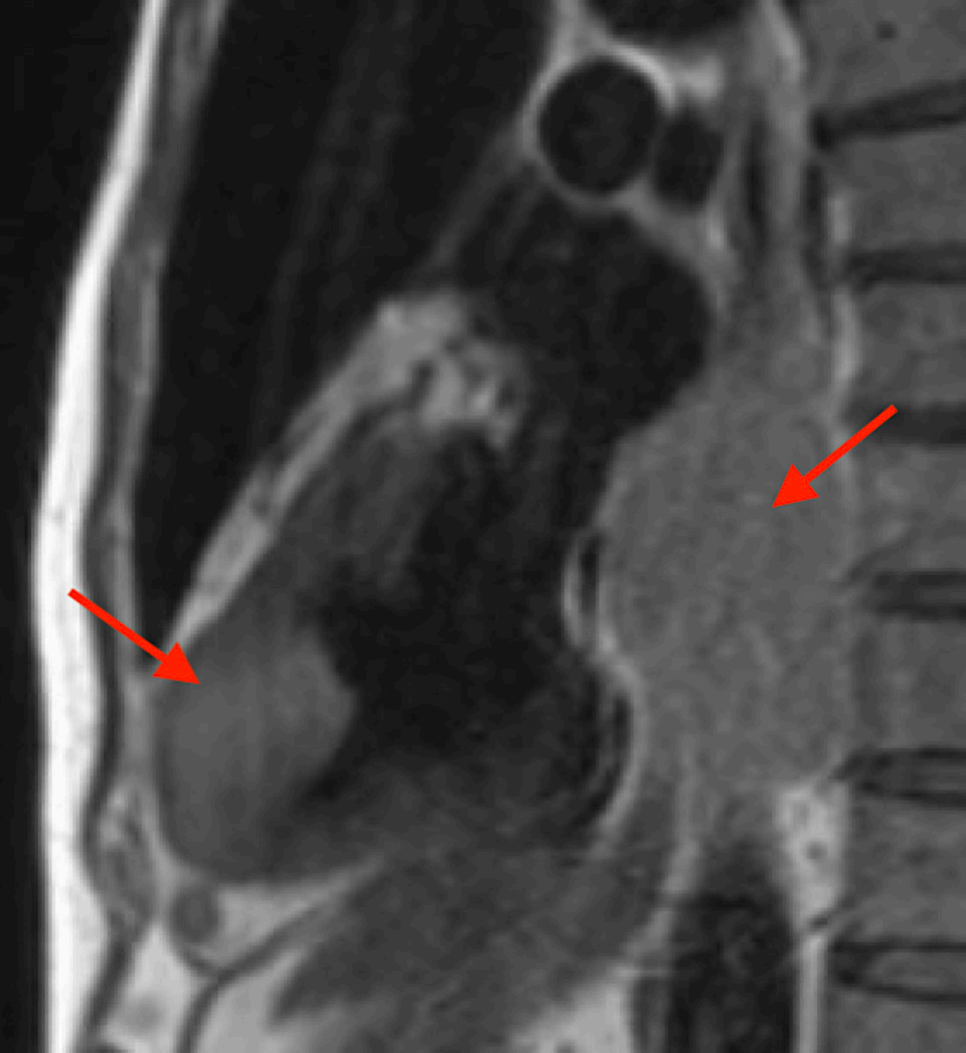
Cardiac MRI T2w TSE (turbo spin echo) pre Gd‐contrast, left ventricle apical and posterior to the LA masses with intermediate signal intensity (arrows).
